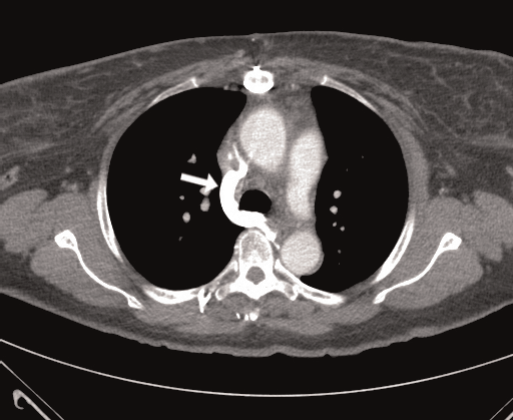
CT scan with contrast shows prominent caliber of the azygous vein due to collateral flow.
